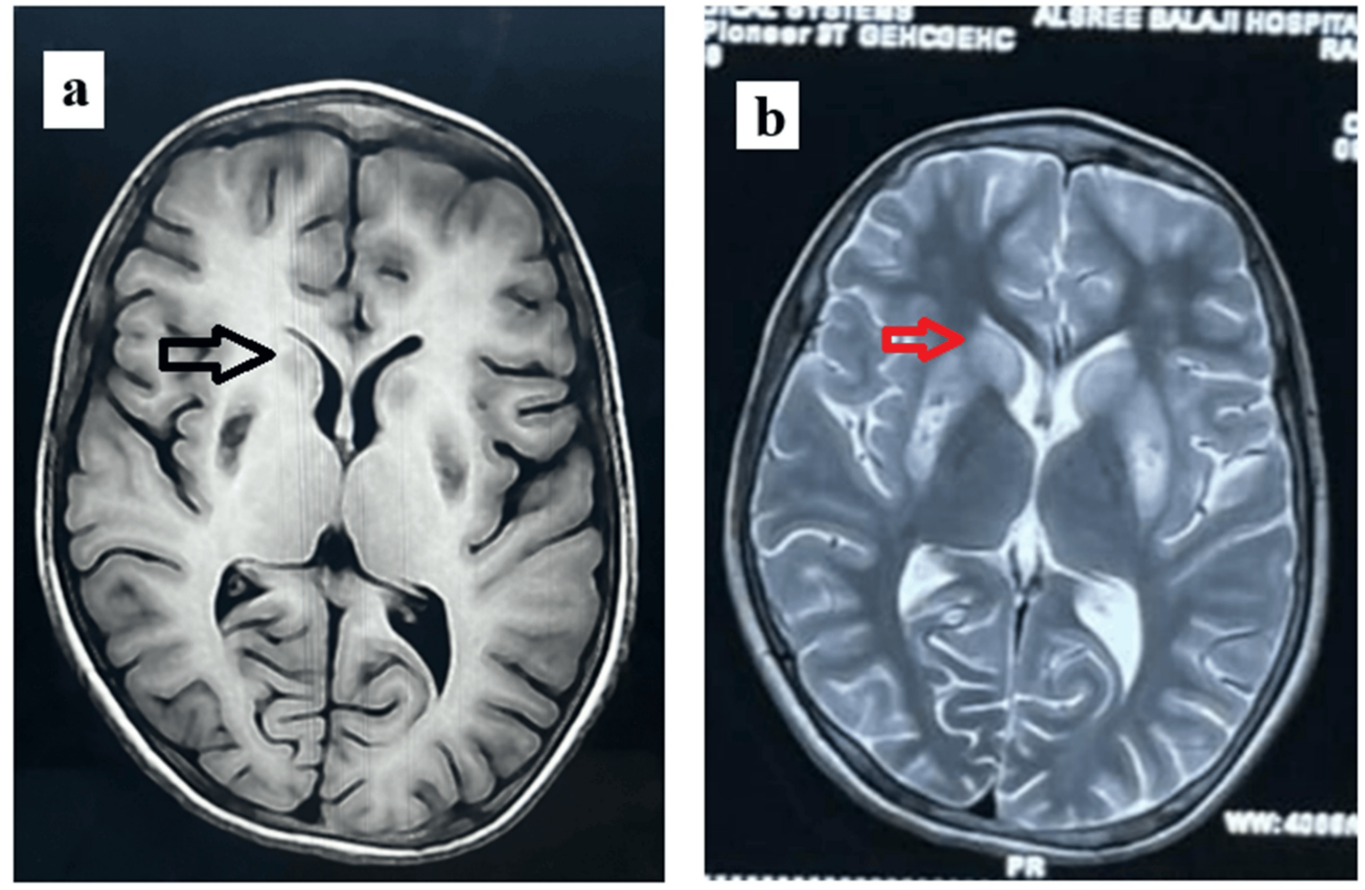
Brain MRI with T1-weighted sequences showing (a) hypointensity (black arrow) and T2-weighted sequences showing (b) hyperintensity (red arrow) of the bilateral putamen and caudate nucleus
